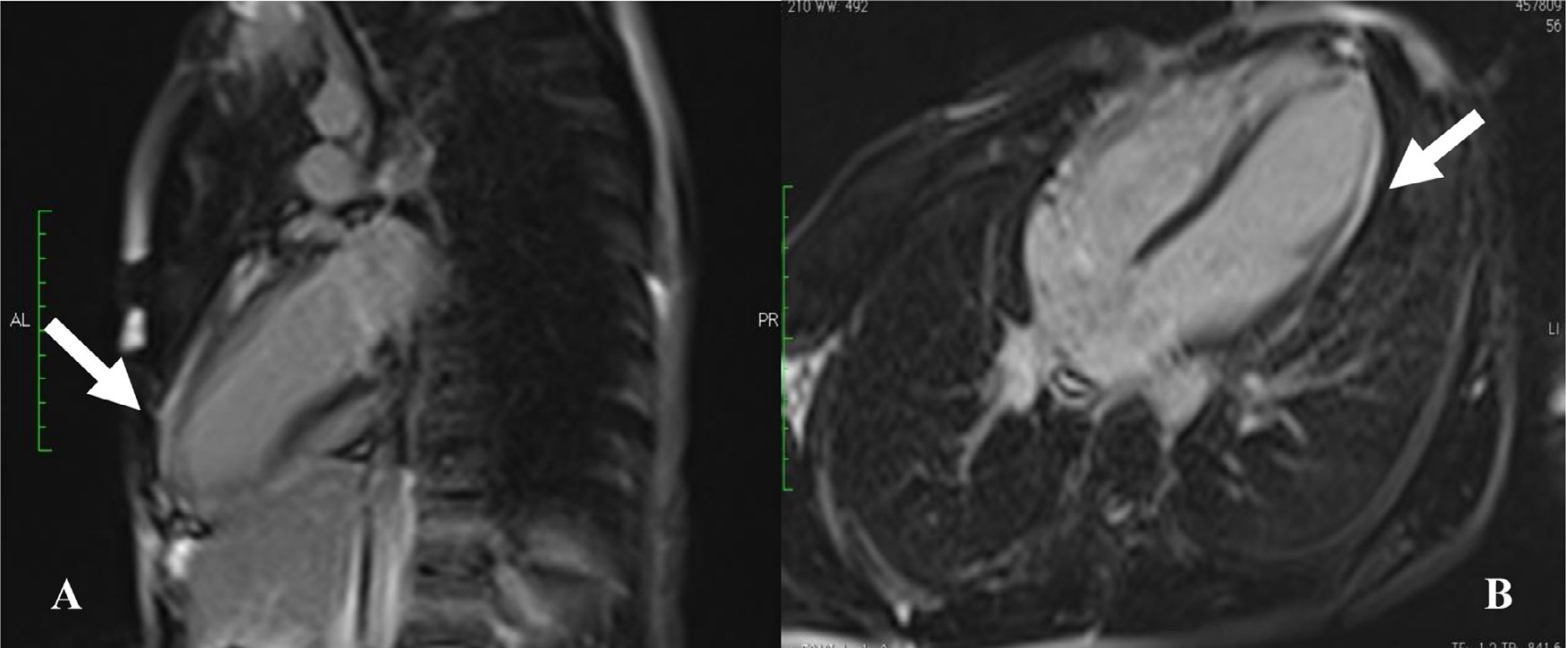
Phase sensitive inversion recuperation MRI sequences revealing late subepicardial enhancement in the form of a hypersignal after injection of gadolinium (white arrow) in the anterior and inferior wall of the left ventricle in the 2-chambers view (Image A); in the lateral wall and apical wall in the 4-chambers view (Image B).
